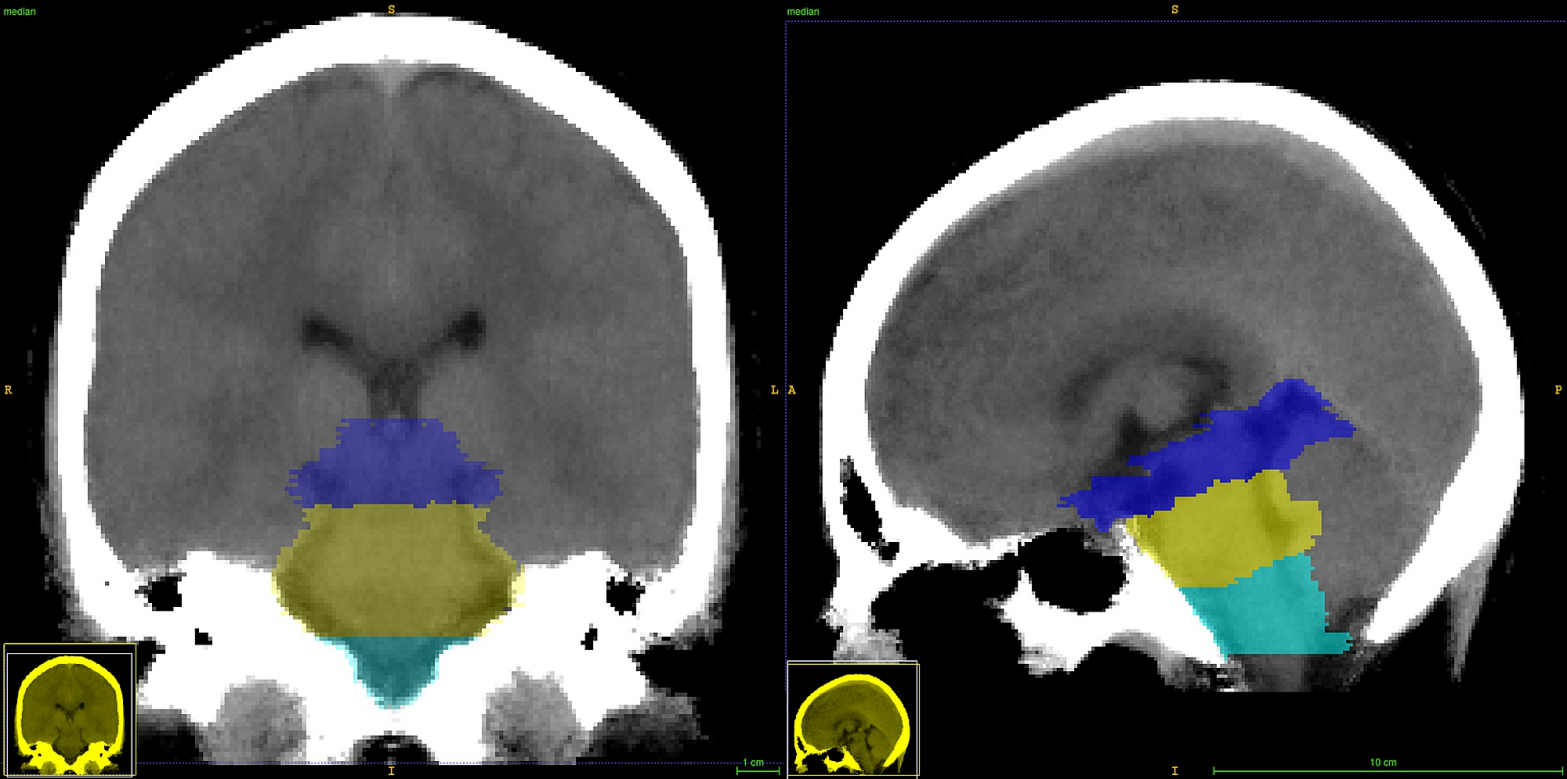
CT template Sagittal and coronal views of the CT template along with the three basal cistern section segmentations: mid-brain (blue) from the superior edge of the quadrigeminal plate to the superior pontine notch, pons (yellow) from the midbrain to the inferior pontine notch, and medulla from the inferior limit of the pons to the superior edge of C1 (turquoise) CT: computed tomography
